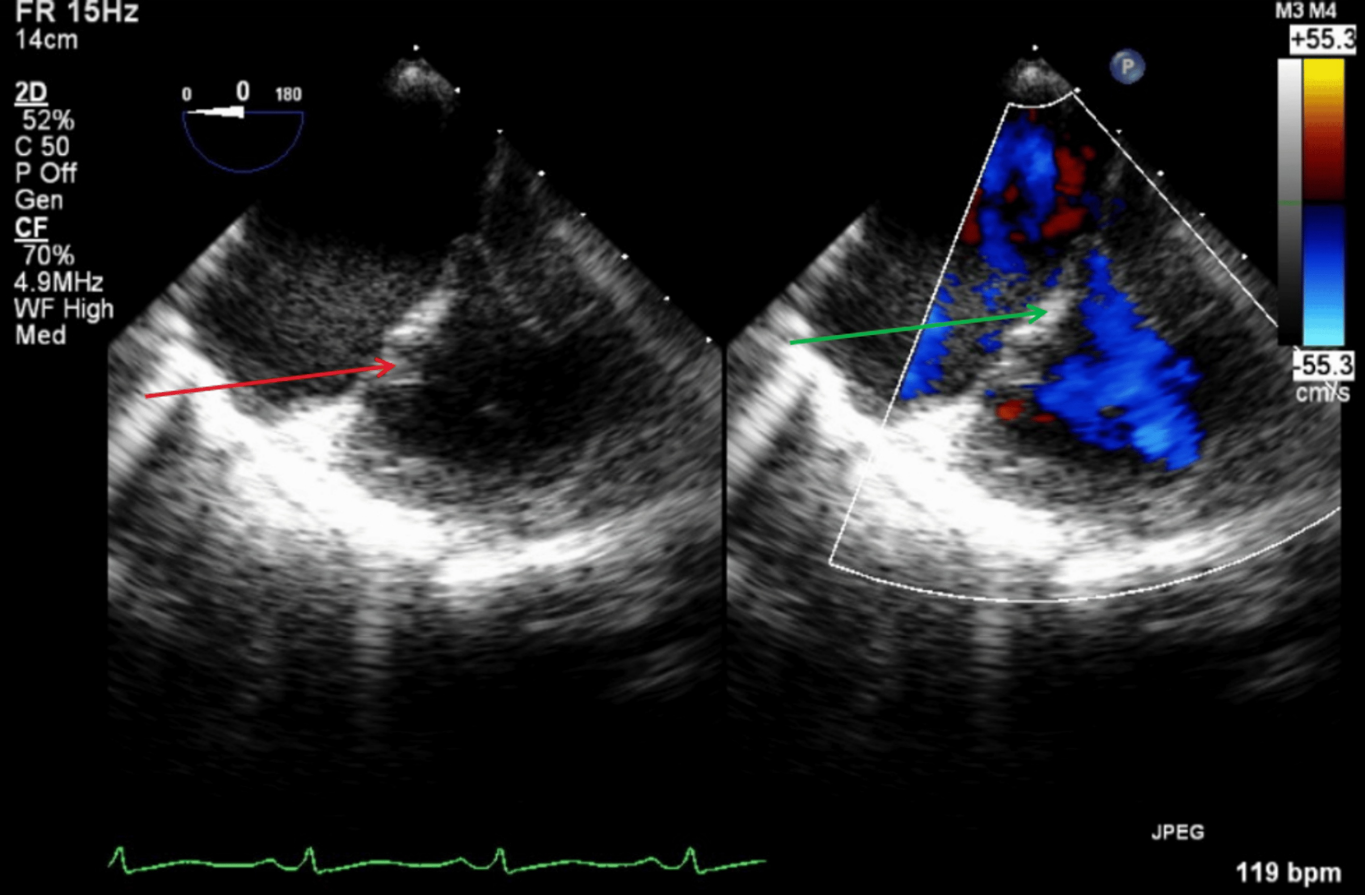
TEE mid-esophageal right two-chamber view with and without color Doppler during systole. The red arrow shows tricuspid valve with no visible vegetations. The green arrow shows no findings of tricuspid valve regurgitation with color Doppler. TEE: transesophageal echocardiography
